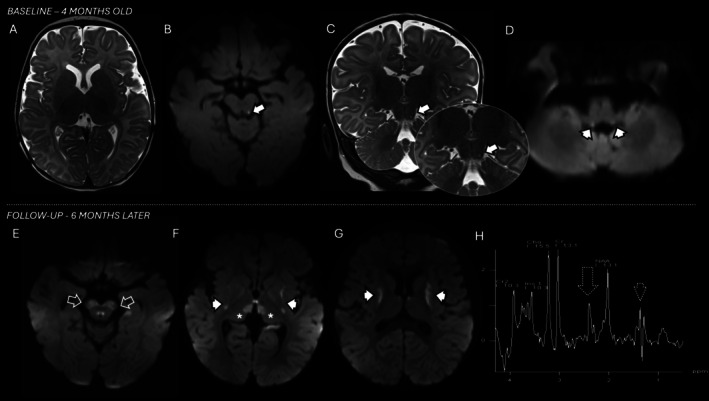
MRI progression for patient #1. Initial MRI brain performed at 4 months of age during presentation for cardiomyopathy and influenza infection (A–D) and follow‐up MRI and MRS (short echo‐time) studies 6 months later (E–H) after seizure onset. Selective lesions were noted at the onset involving the left inferior colliculus with reduced diffusivity (ADC MAP not shown) and T2 hyperintensity (arrows, B and C) and with reduced diffusivity along the vestibular nucleus in the inferior cerebellar peduncles (arrowheads, D). Progression with new lesions with reduced diffusivity (ADC MAP not shown) were noted in the follow‐up study including brainstem (open arrows, E), particularly in the cerebral peduncles and oculomotor nuclei, as well as in the mediodorsal aspect of the thalami (asterisks, F) as well as in the putamen bilaterally (arrowheads, F and G). Abnormal peaks of succinate and lactate are noted resonating at 2.36 and 1.3 ppm (dotted arrow and dotted arrowhead, H).
